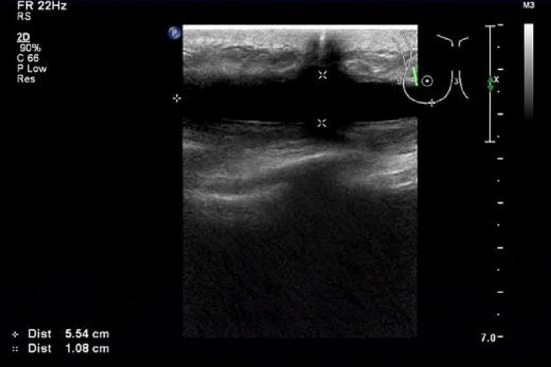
Ultrasound image of seroma after removal of the drains in a breast cancer patient who underwent MRM on the right side
